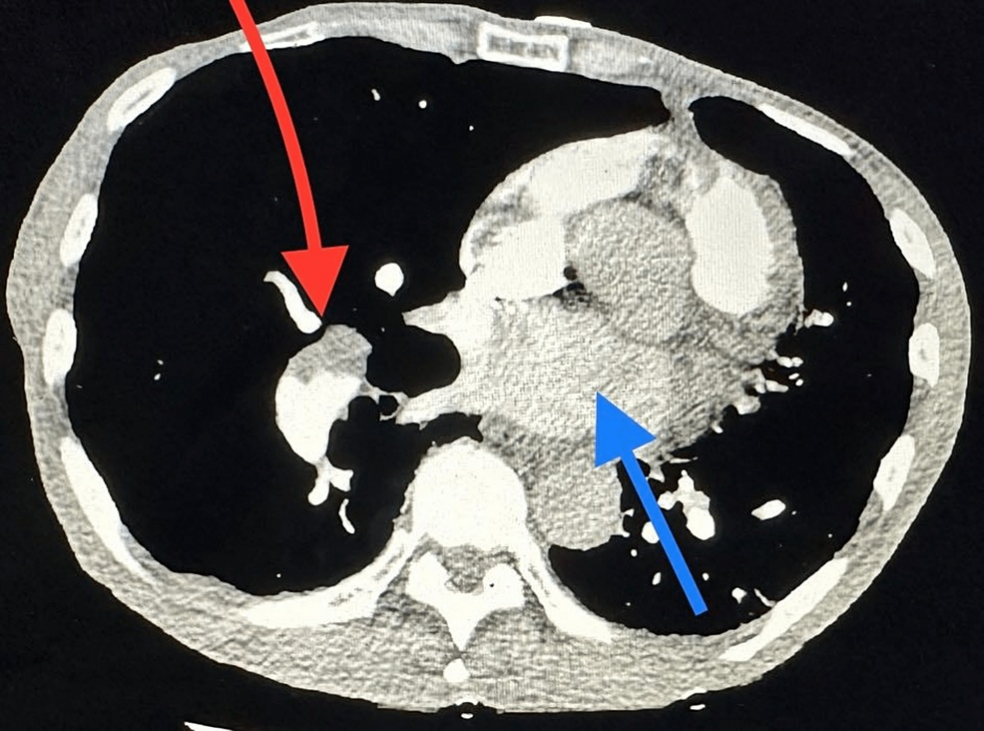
CTA chest showing mural thrombus in the right lung (red arrow) Dilated pulmonary artery (blue arrow) consistent with pulmonary artery hypertension. CTA: computed tomography angiography.
